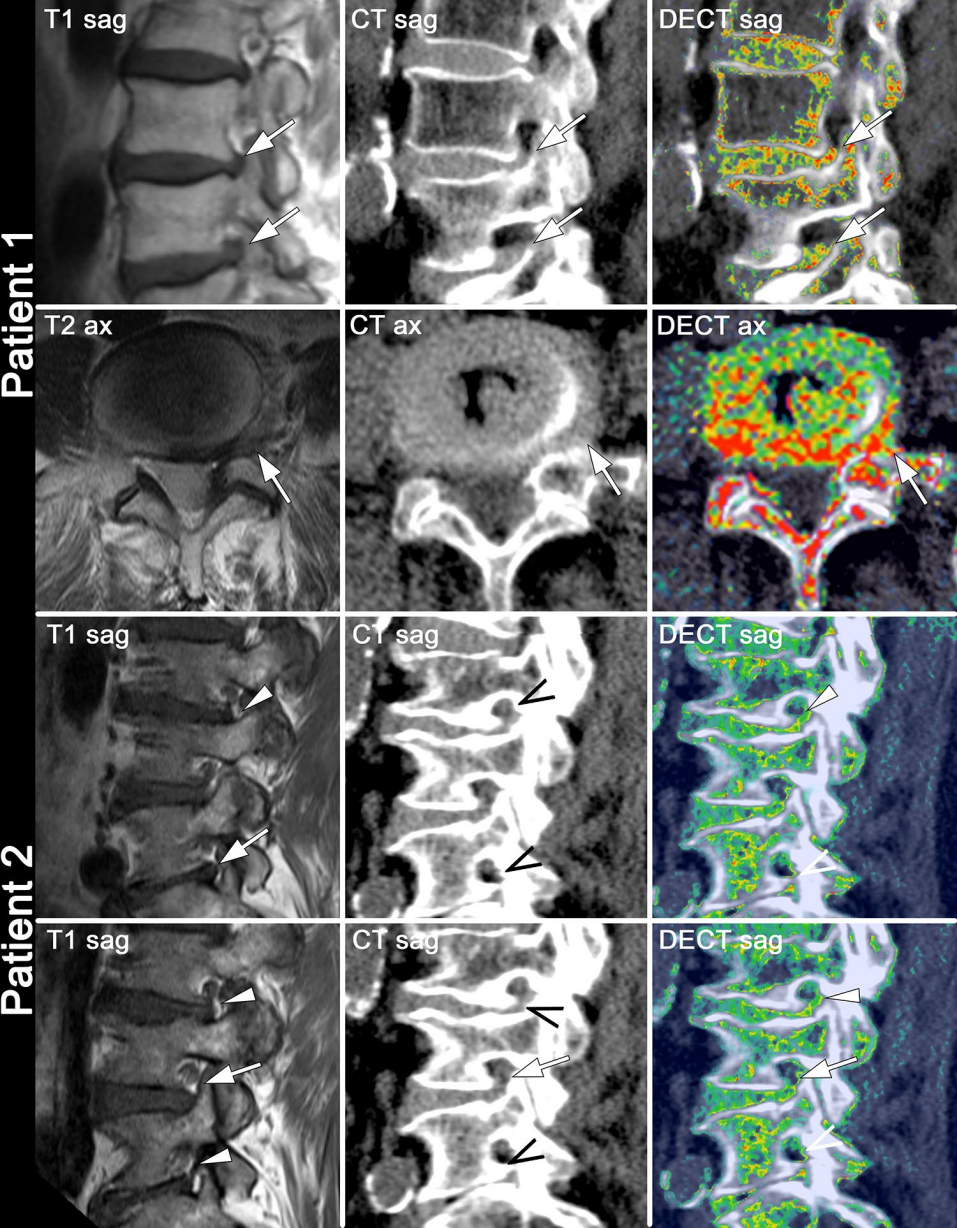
Imaging examples from two different patients. For each patient (left to right) MRI, standard CT, and collagen-sensitive dual-energy reconstructions are shown with the according consensus scoring. Arrows: discoid foraminal stenosis; arrowheads: mixed discoid/osseous foraminal stenosis; open arrowheads: osseous foraminal stenosis.
